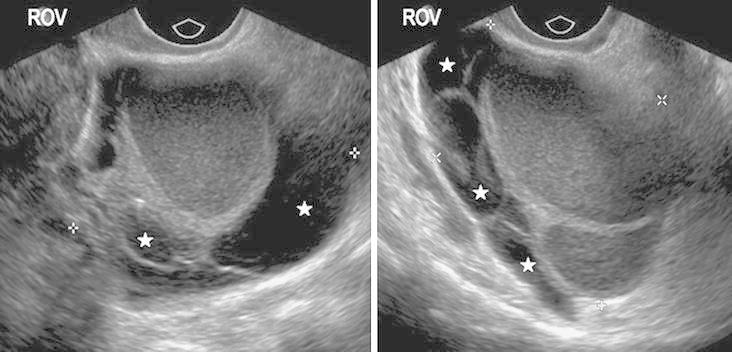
Transvaginal ultrasound images showing typical features of a right ovarian endometrioma (ROV): multiloculated cystic structure, uniform low-level internal echoes with peripheral “candle wax” appearance due to repeated hemorrhage of variable age (*filled star*).
